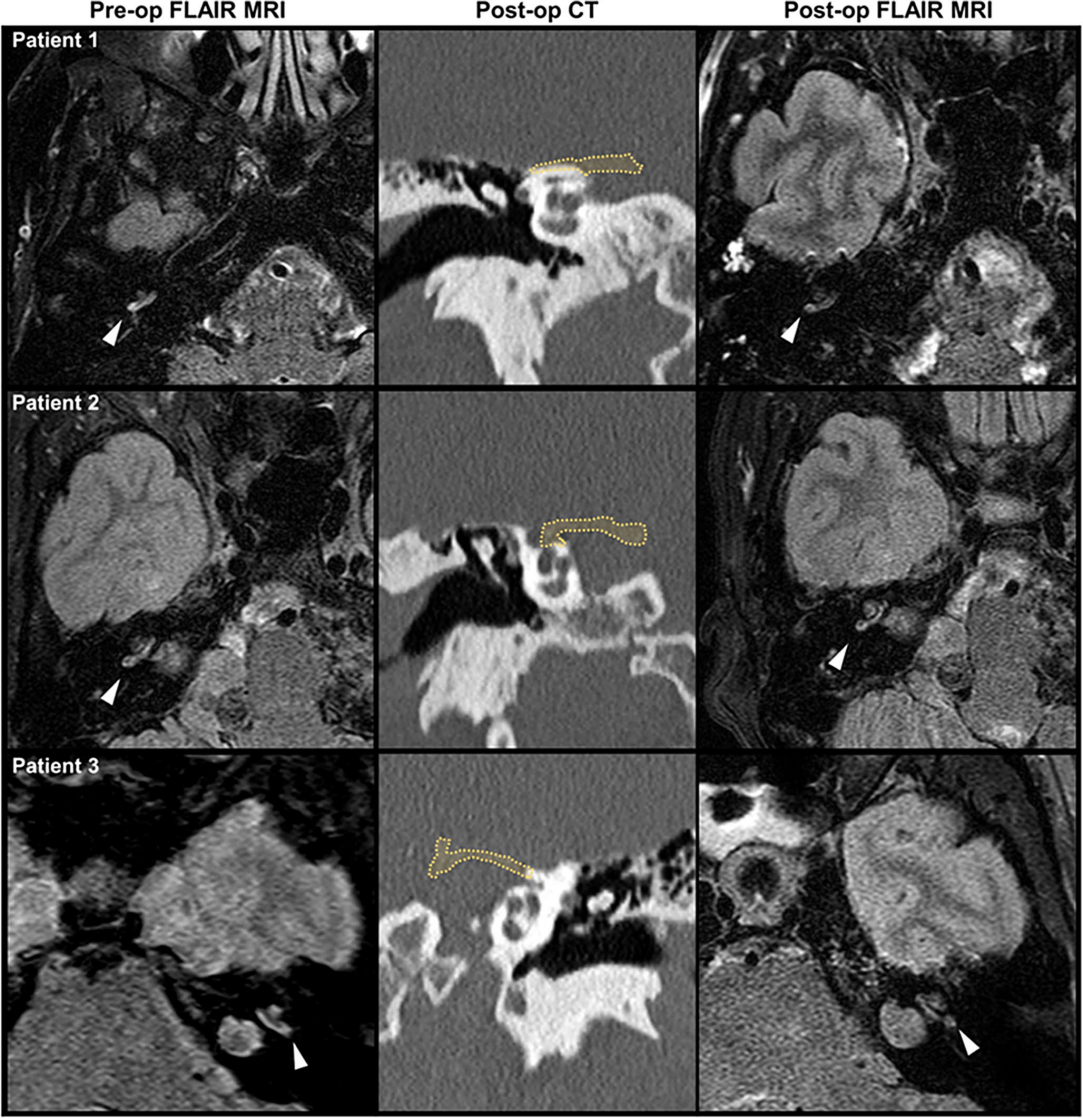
Middle fossa decompression does not reverse intralabyrinthine FLAIR hyper-intensity at one year follow-up. Pre-contrast axial FLAIR MRI images were obtained one to seven days prior to middle fossa decompression (left column) and one year following surgery (right column). Sections are through the basal turn of the cochlea (denoted by white arrowhead). Post-operative images show persistence of the FLAIR signal hyper-intensity within the basal segment of the cochlea in all three patients. The middle column represents post-operative coronal CT temporal bone through the cochlea and head of malleus. The yellow dashed line denotes the area of bone removed during the middle fossa decompression
